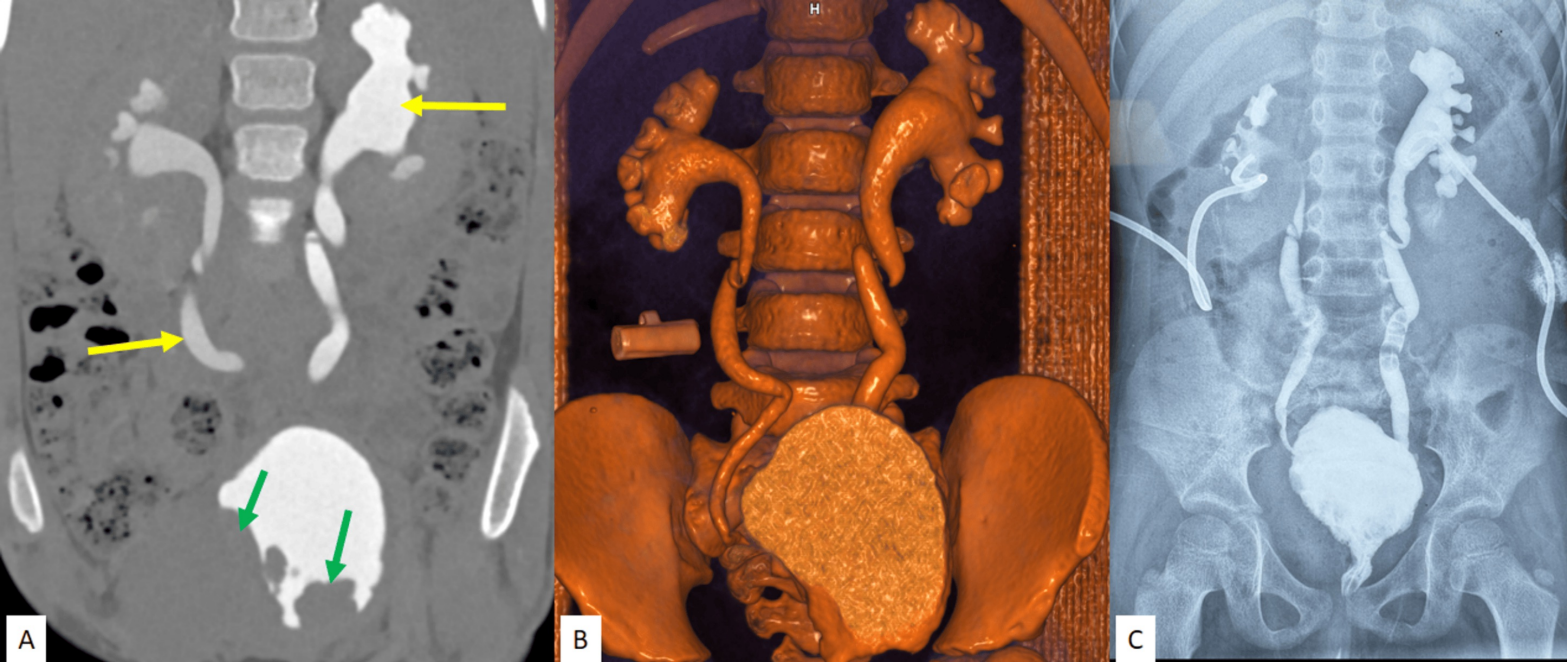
(A) Excretory phase of CECT scan (coronal section) showing a filling defect in the bladder lumen (green arrows) and contrast retention in bilateral dilated pelvicalyceal systems and ureters (yellow arrows). (B) 3D VRT image demonstrating bilateral moderate hydroureteronephrosis. (C) Bilateral percutaneous nephrostogram images. CECT: contrast-enhanced computed tomography, VRT: volume-rendered technique
